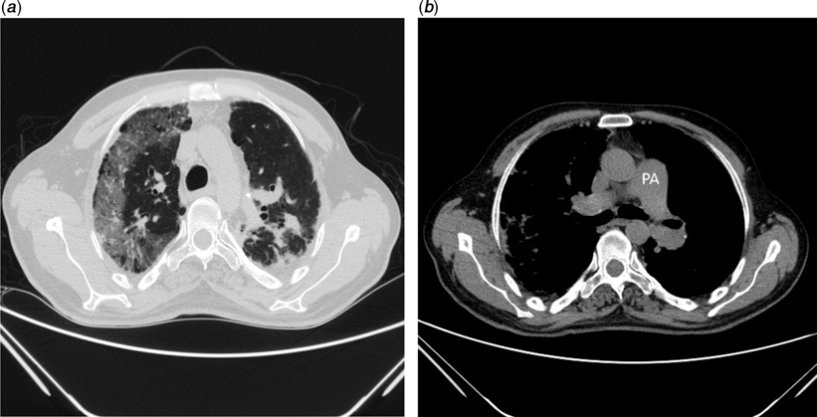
Chest CT scan shows (*a*) ground-glass appearance and peripheral round opacity; (*b*) dilation of pulmonary artery.
